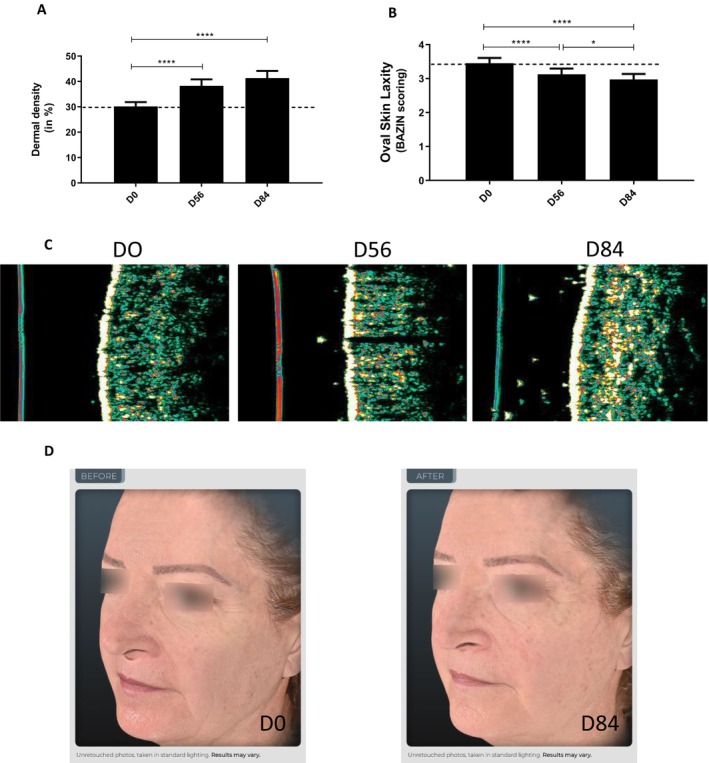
Clinical evaluation of the product with 1% TFC‐1326 on dermal density and skin laxity. Density of the dermis (A) was evaluated by ultrasound measurements, and skin laxity of the face oval (B) was assessed by Bazin scoring. Results are expressed as means ± SEM, *N* = 20. p* < 0.05, *p* < 0.0001. Representative ultrasound images of dermis density (C) and representative photographs of face sagging (D) from one woman (subject *n*°12) before (D0), during (D56), and after (D84) the topical application with 1% TFC‐1326.
